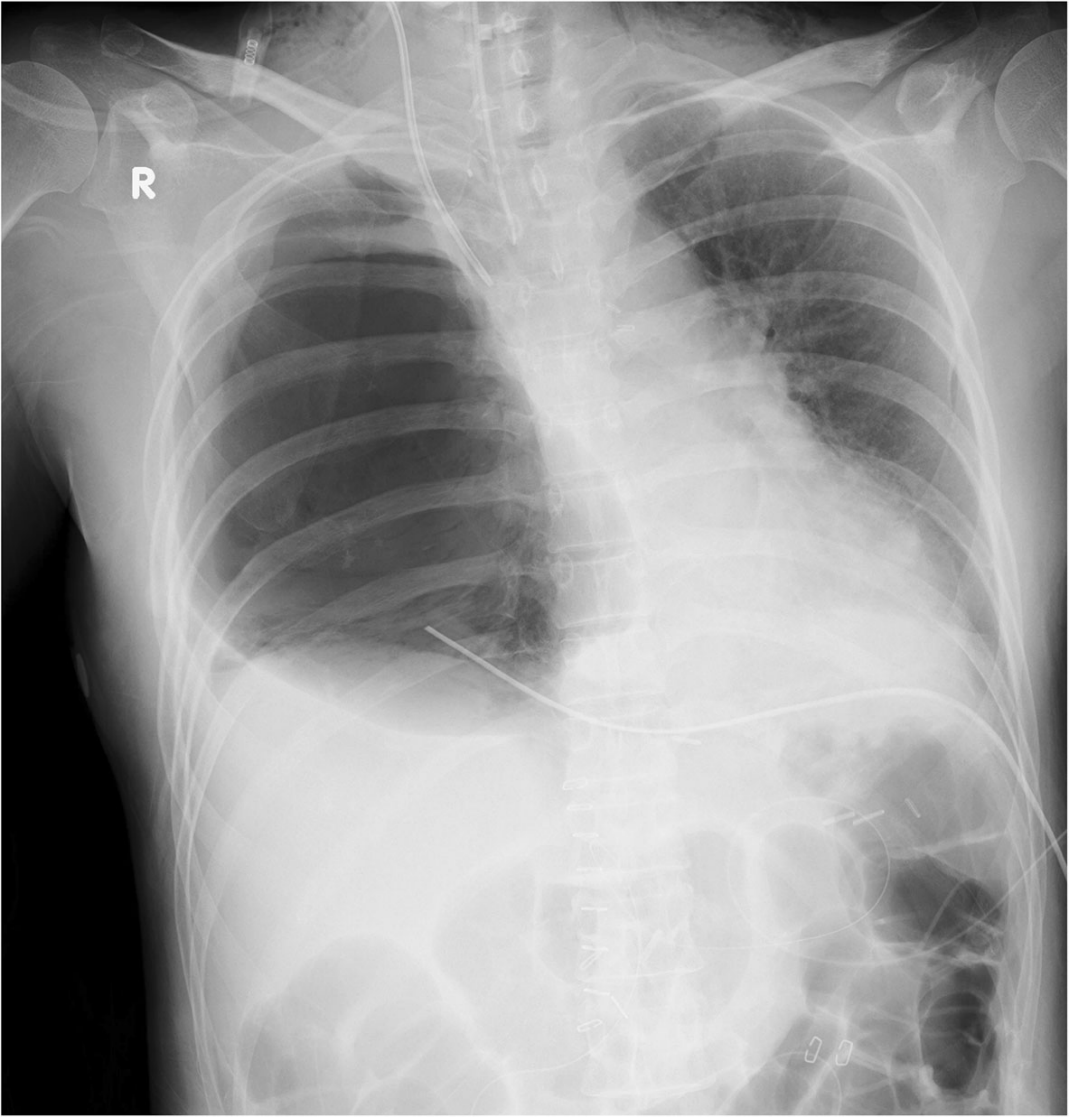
Chest radiograph obtained upon rapid development of tachypnea
